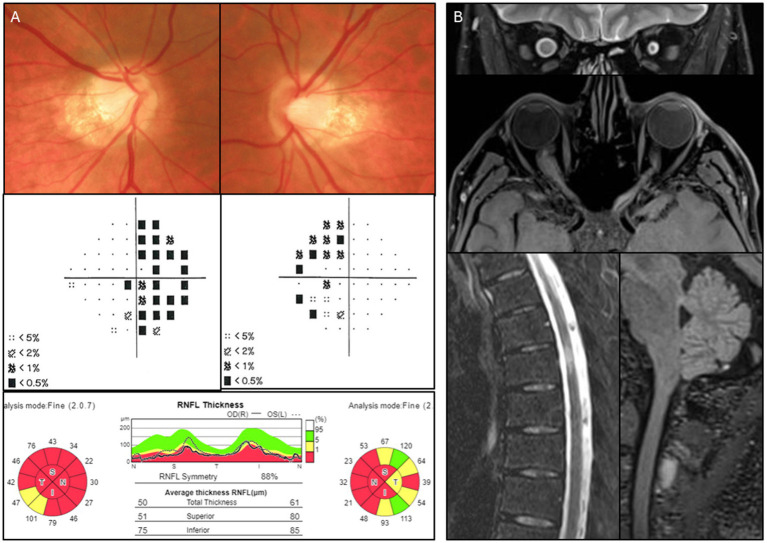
(A) Ophthalmological findings: in the upper panel fundoscopy shows small and tilted optic discs, with diffuse pallor of the right optic disc and temporal pallor of the left optic disc; in the middle panel VF show bitemporal hemianopia (OD > OS); in the lower panel OCT shows diffuse right generalized thinning of the RNFL and left optic bowtie atrophy. (B) Ophthalmological findings: in the upper panel fundoscopy shows small and tilted optic discs, with diffuse pallor of the right optic disc and temporal pallor of the left optic disc; in the middle panel VF show bitemporal hemianopia (OD > OS); in the lower panel OCT shows diffuse right generalized thinning of the RNFL and left optic bowtie atrophy. (B) Radiological findings: in the upper panel coronal T2-weighted brain MRI shows right optic nerve glioma and axial T1-weighted Fat-Saturated brain MRI shows chiasmal atrophy and right optic nerve glioma; in the lower panel sagittal STIR spinal cord MRI shows a demyelinating lesion in the dorsal spinal cord and sagittal FLAIR brain MRI shows a neurofibroma posterior to the C3 lamina.
